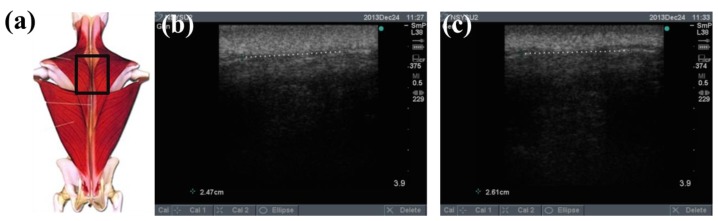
Results from ultrasound images: (a) measured trapezius position; (b) preparatory action; (c) anteflexion action.
